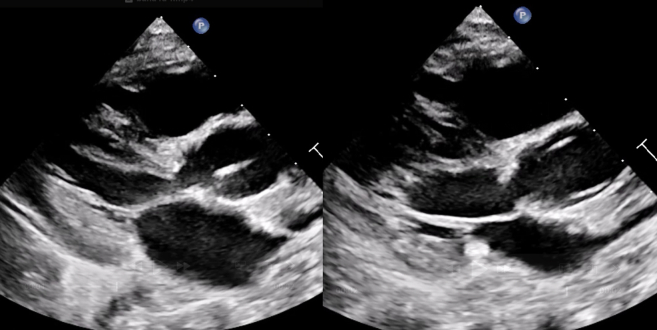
Postoperative Transthoracic Echocardiogram, Parasternal Long Axis View, Showing Newly Identified Subaortic Stenosis in Systole and Diastole
